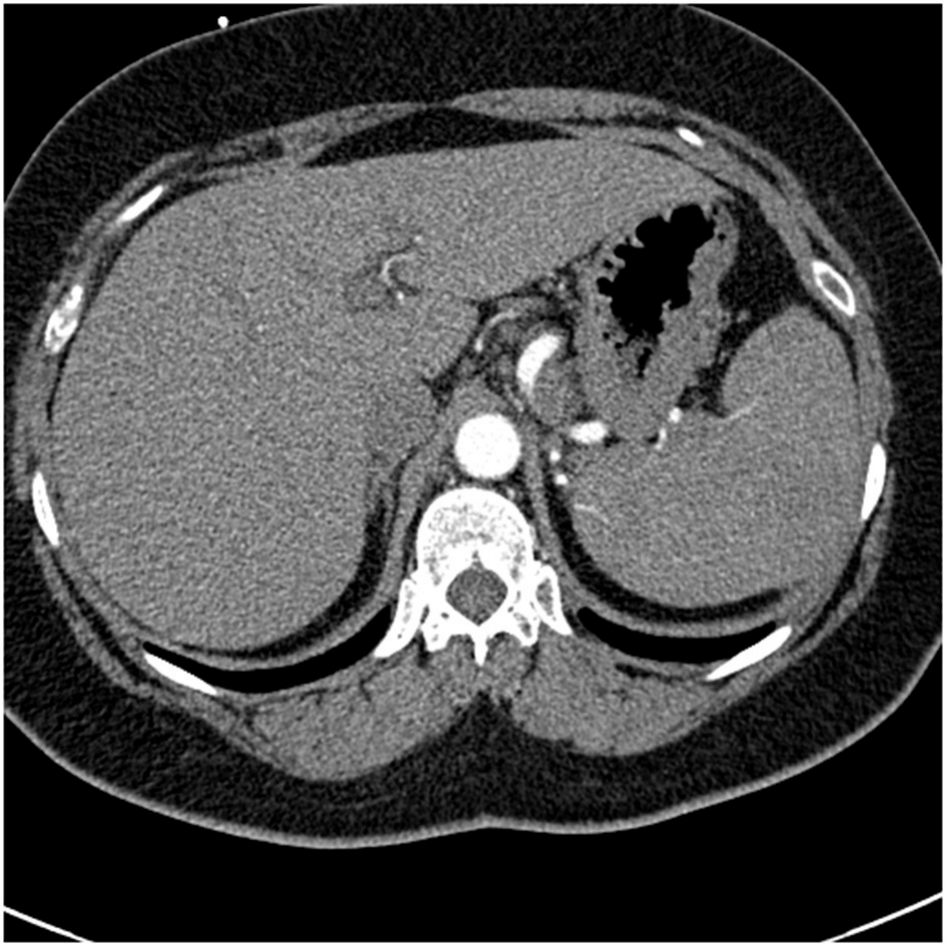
A lower chest CT scan image from the same scan as Figure 5 showing a mildly enlarged spleen, which is a common feature with common variable immunodeficiency.
